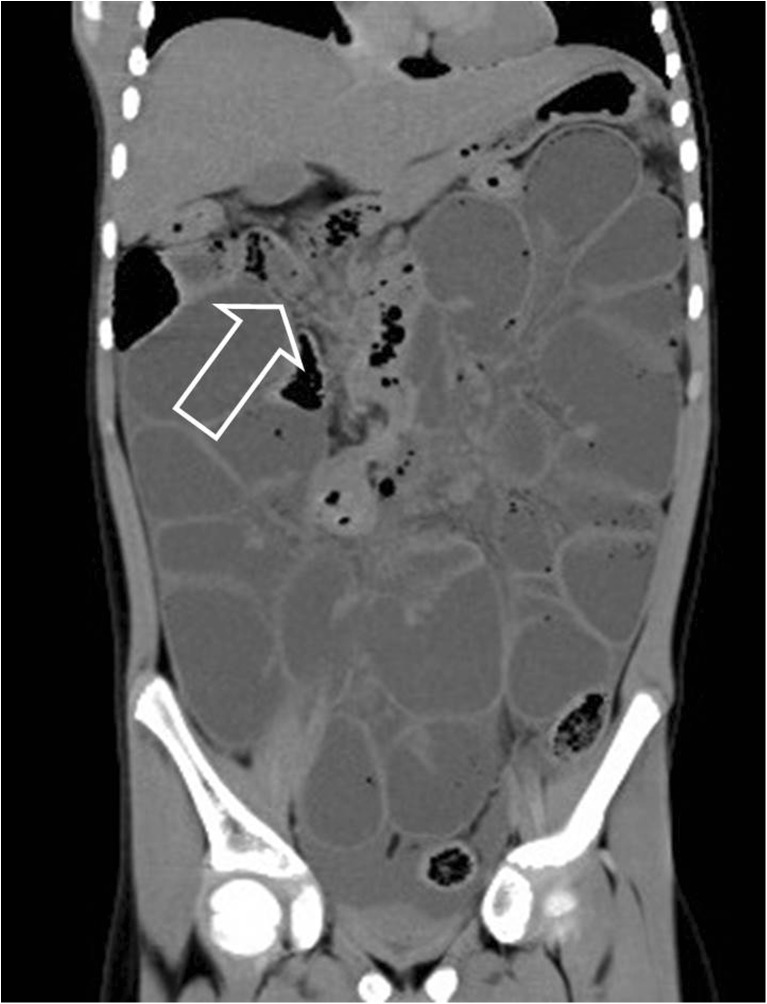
Postmortem CT in a girl age 6 years 11 months with an adhesion ileus. Coronal reconstruction shows the presence of collapsed small-bowel loops in the right upper abdomen (*arrow*). On autopsy an adhesion ileus was confirmed
